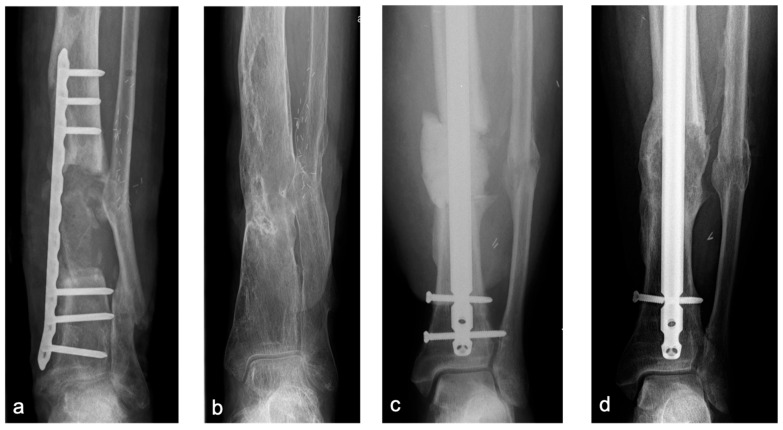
Case 1 (a,b): Bone defect of the distal tibia (7 cm), reconstruction using the BBT (a): postoperative X-ray, (b): at 10-year follow-up. Case 2 (c,d): Bone defect of the distal tibia (4 cm), Step I of the BBT (c): postoperative X-ray with temporary intramedullary nail osteosynthesis and PMMA spacer; (d): at 4-year follow-up after Step II of the BBT.
